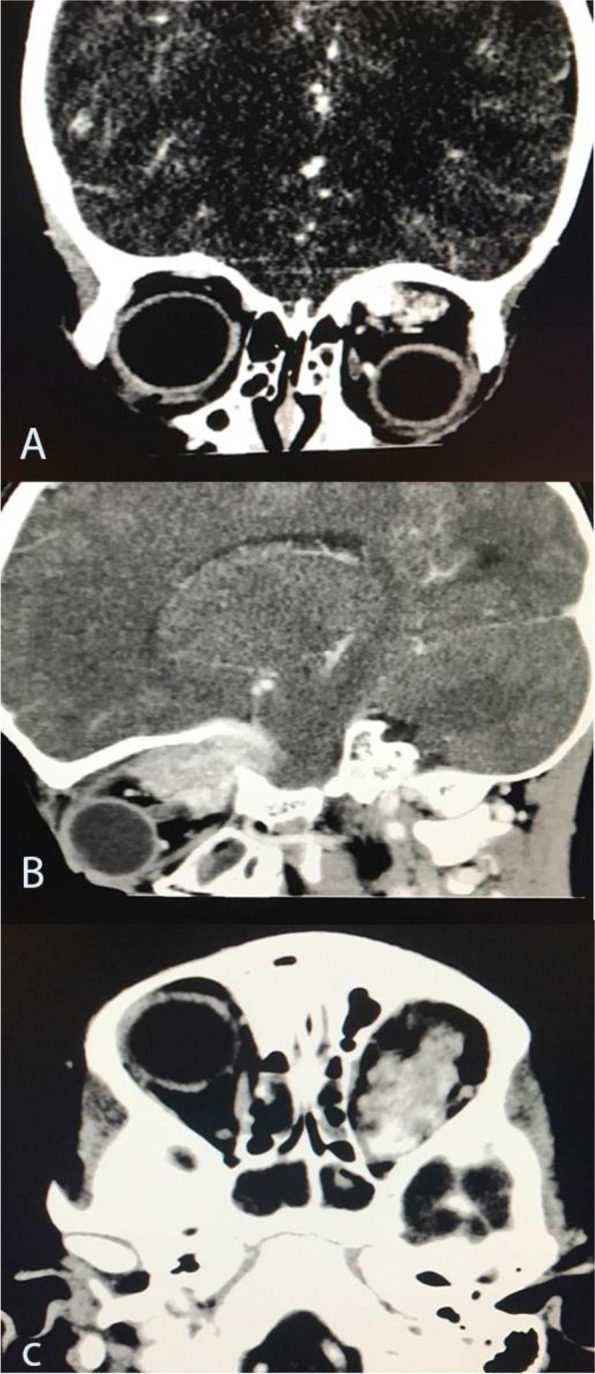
Orbital CT scan with coronal (A), sagittal (B) and, axial views (C) showing well demarcated mass along the left superior rectus muscle
